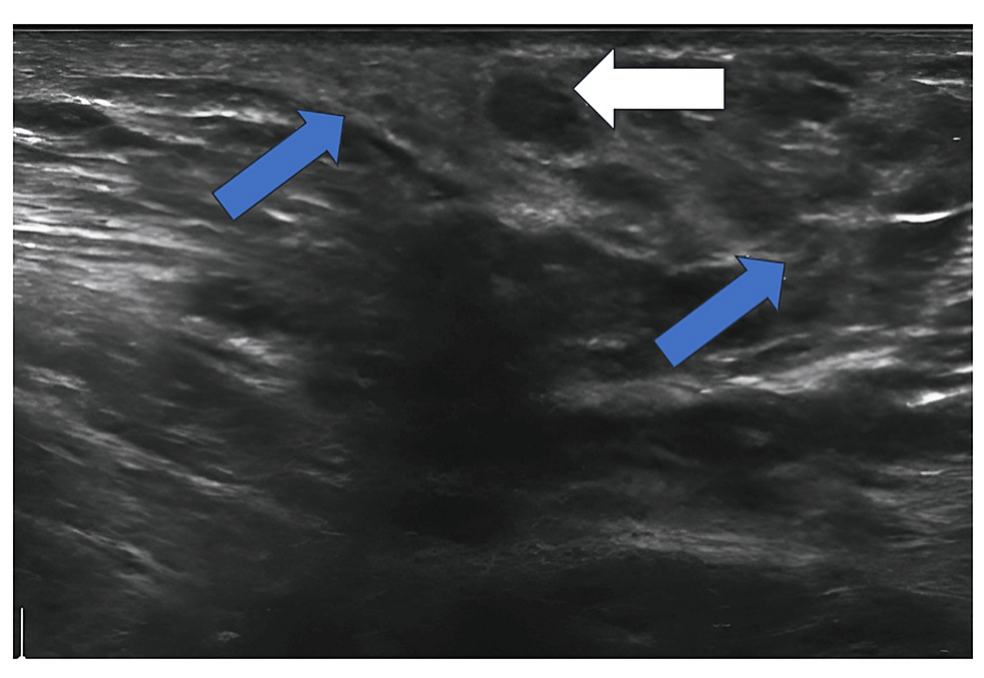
Ultrasonography of the breast tissue. The blue arrows show fibrous echogenic stroma; the white arrow shows hypoechoic glandular tissue.
